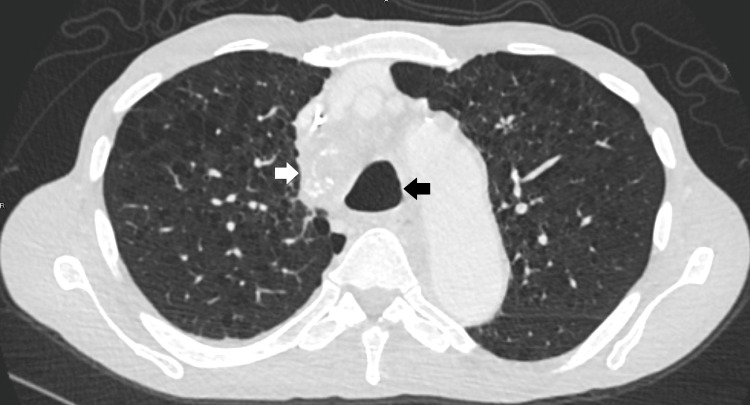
Repeat CT scan after four cycles of chemotherapy and the removal of tracheal stent shows dramatic reduction in mediastinal mass (white arrow), as well as the resolution of tracheal compression (black arrow).
